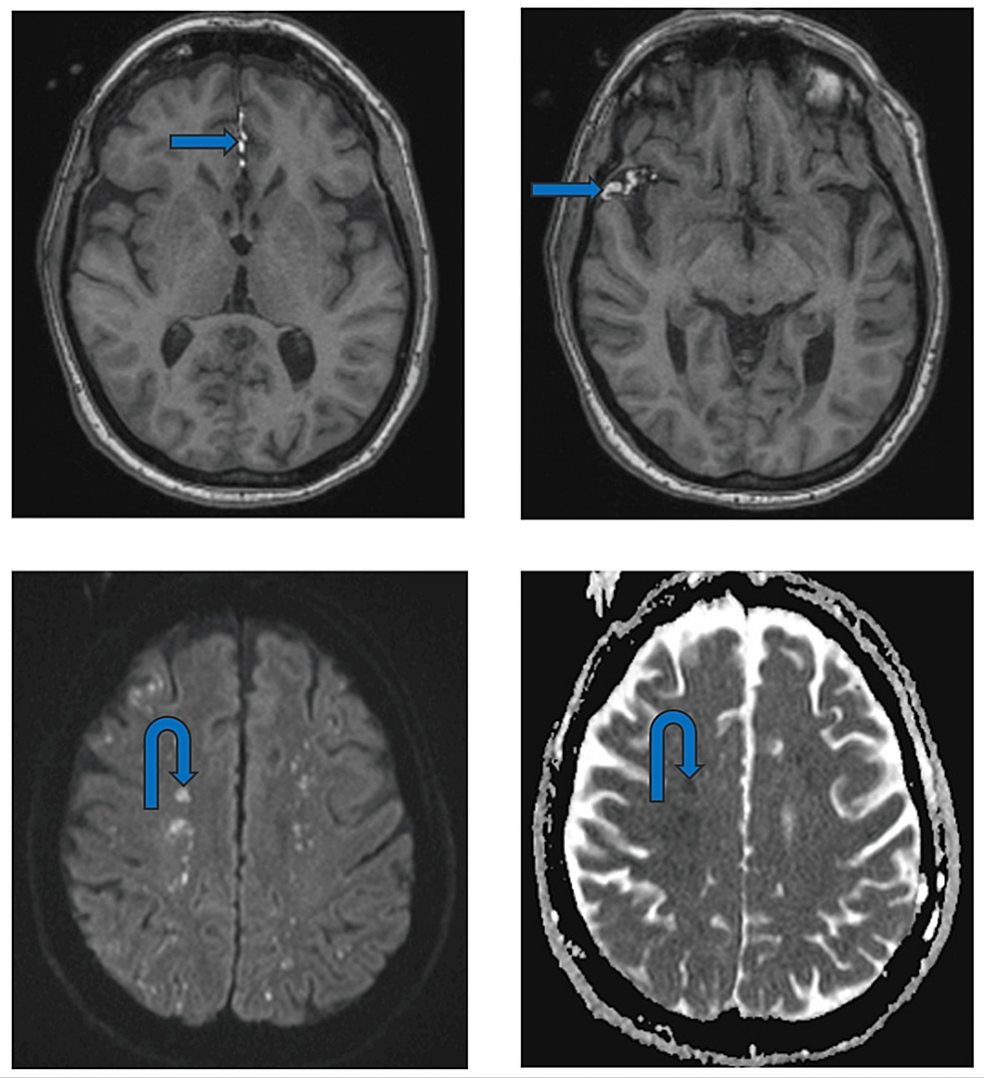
Axial MRI T1 images of the brain showing high signal fat in the sylvian fissure and interhemispheric area (arrows). DWI and ADC images showing changes in watershed regions (curved arrows).
